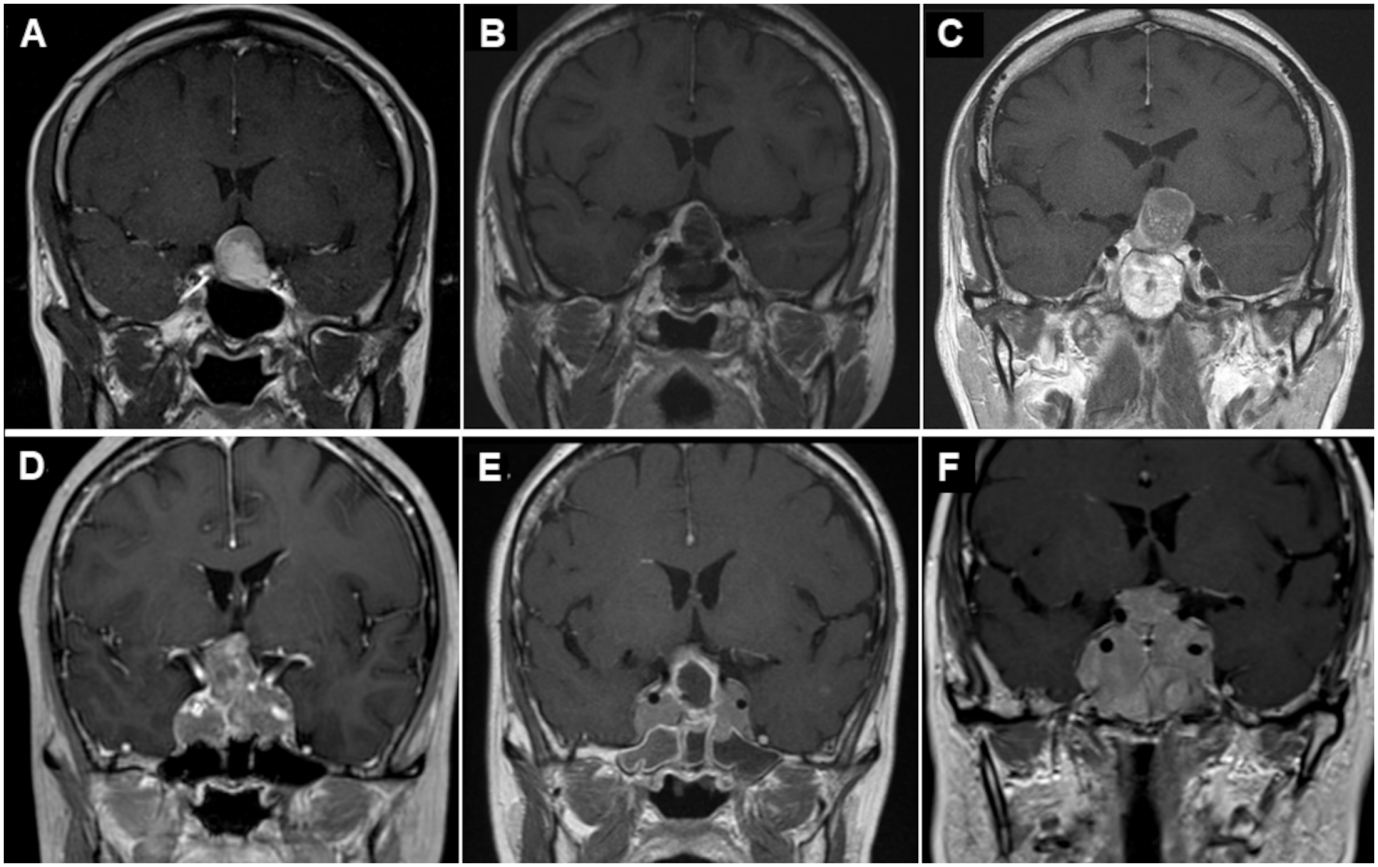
Representative case of regrowing nonfunctioning pituitary tumors after prior TSS. GTR case (A–C) and PR case (D–F). Preoperative (A) and postoperative (B) enhanced T1-weighted MR images showing GTR after initial surgery. However, enhanced T1-weighted MR image before reoperation (C) showing tumor regrowth and compression of the optic nerve again 137 months after the primary surgery. Preoperative (D) and postoperative (E) enhanced T1-weighted MR images showing PR after initial TSS. Preoperative enhanced T1-weighted MR image before reoperation (F) showing tumor regrowth and compression of the optic nerve again 80 months after the primary surgery. TSS, transsphenoidal surgery; GTR, gross total resection; PR, partial resection; MR, magnetic resonance.
